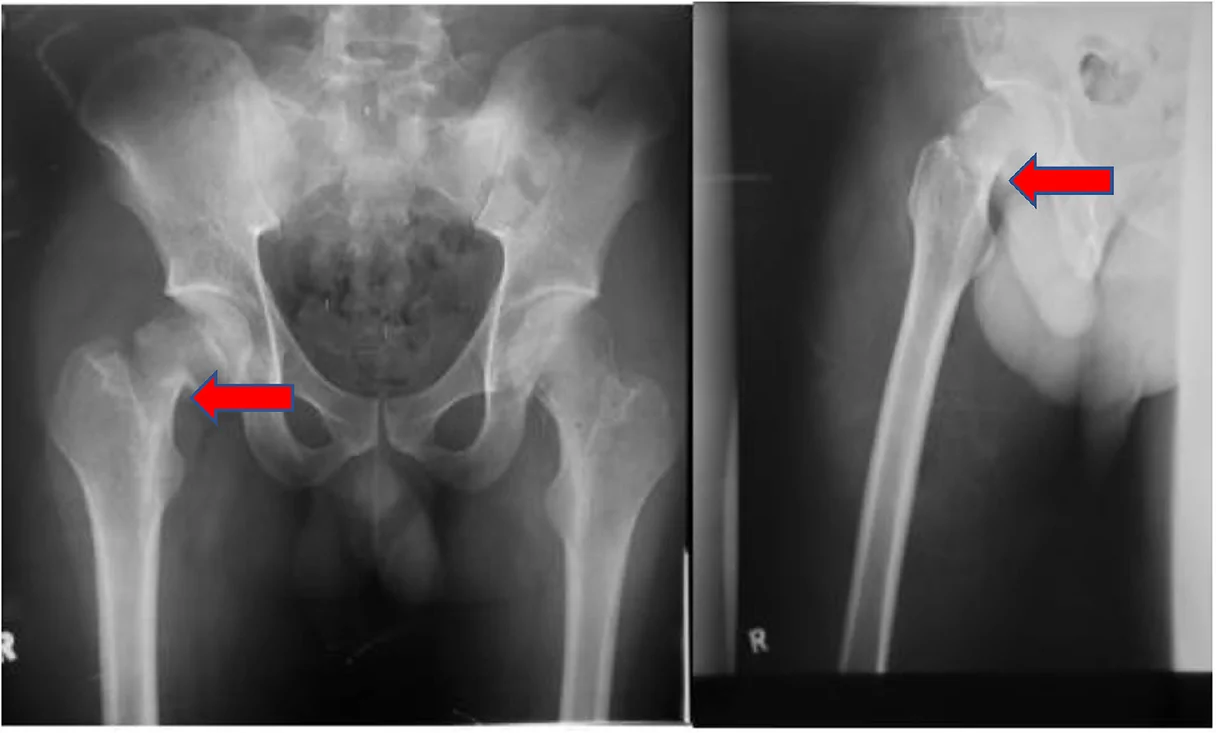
Plain radiograph of pelvis AP and right hip lateral view showing right neck of femur fracture.
